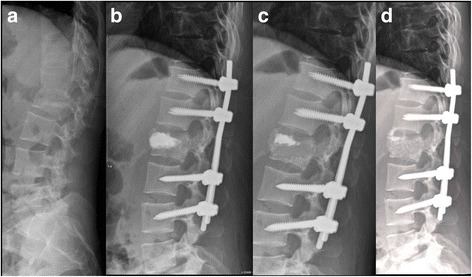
Radiographic image of one patient who received kypoplasty and long instrumentation. a. Lateral roentgenogram of a 25-year-old female patient showing a burst fracture of L2; the kyphotic angle is 30°. b. Postoperative lateral X-ray 3 days after surgery showing excellent restoration after kyphoplasty and long instrumentation; the kyphotic angle is −6°. c. Intact implant and kyphotic angle is −3° three months after surgery. d. Solid anterior fusion (continuous trabeculae of bridging anterior osteophytes without halo signs of screws) was achived and kyphotic angle was 0° 12 months after surgery
